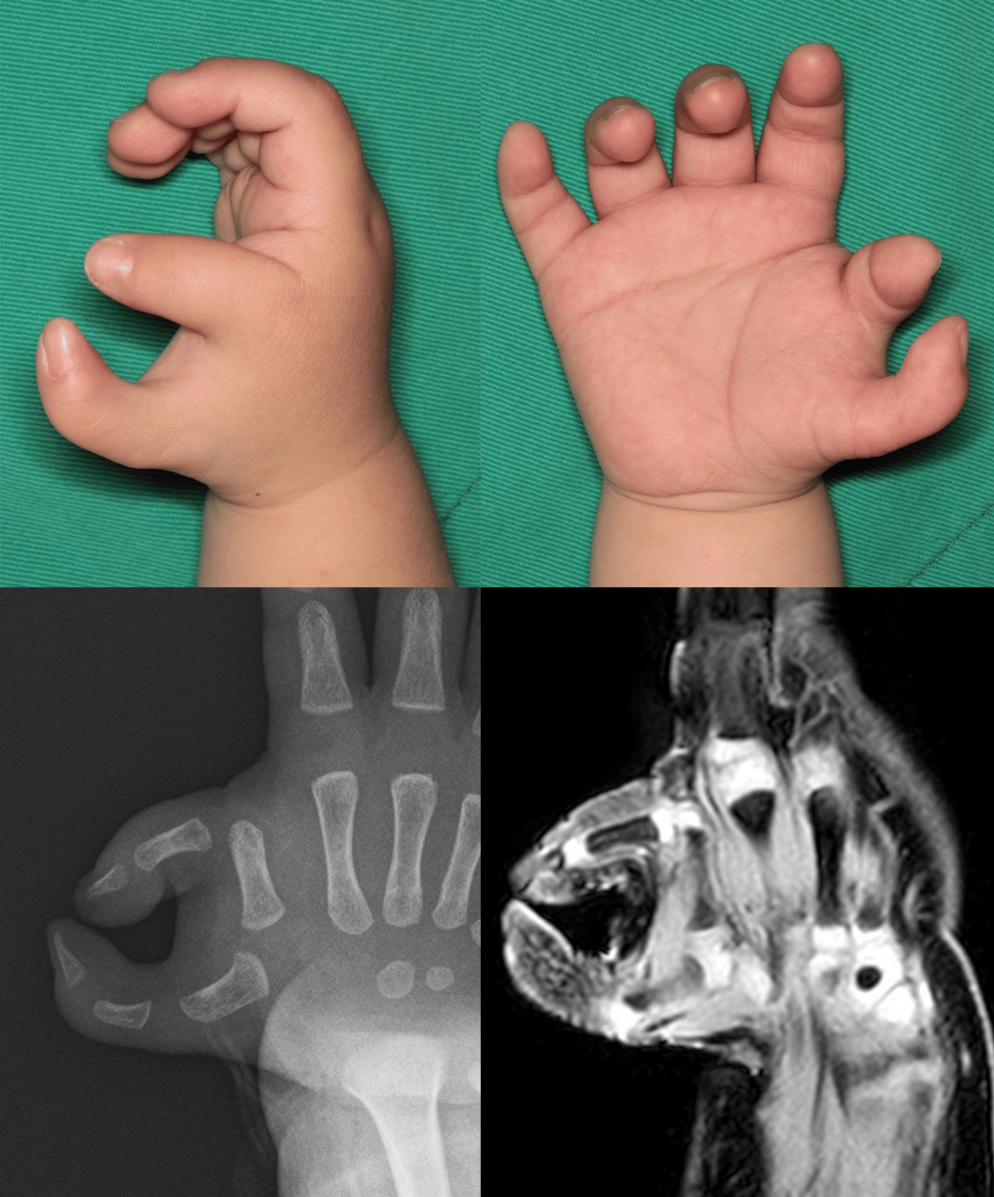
Preoperative findings. A one-year-old boy with Wassel type VI polydactyly. Both the radial and ulnar digits showed severe lateral instability at the metacarpophalangeal (MCP) joint. Preoperative MRI confirmed that the MCP joint structure of the ulnar digit was intact.
